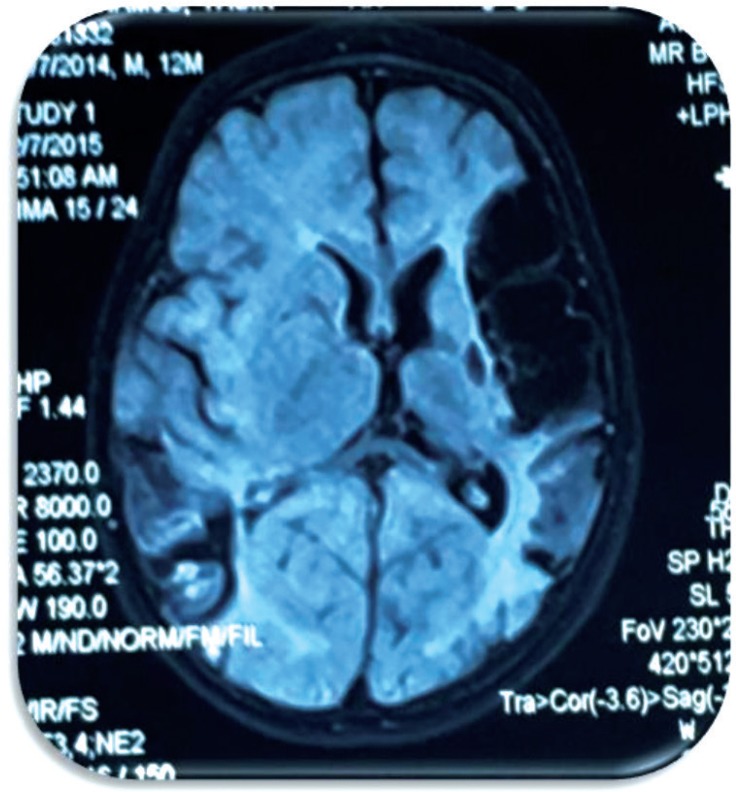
FLAIR sequencing shows hypointensity in left temporoparietal region
